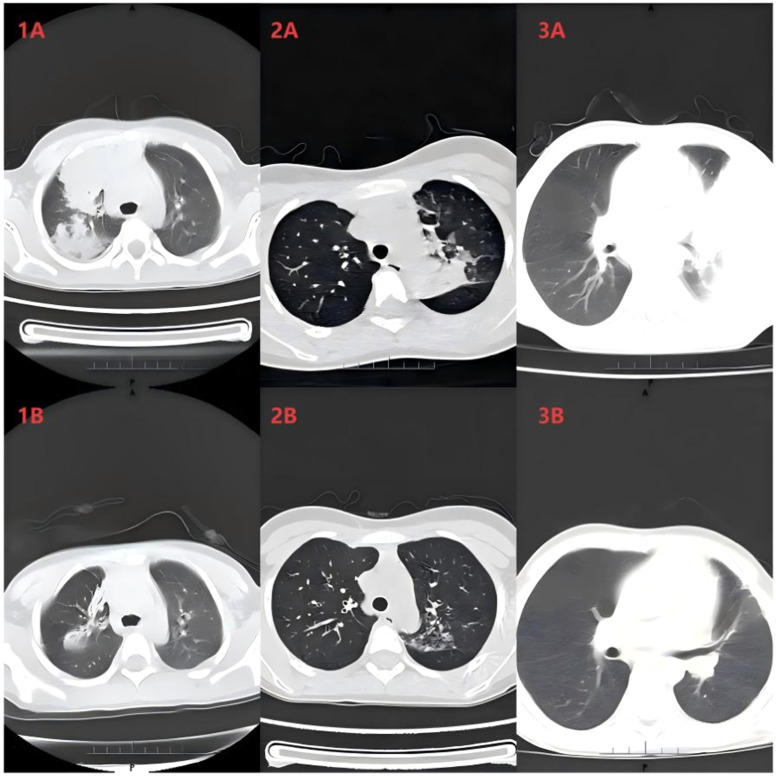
Findings on chest computed tomography before and after BAL. (1A) Case 1 (pre-BAL). (1B) Case 1 (2 weeks post-BAL). (2A) Case 2 (pre-BAL). (2B) Case 2 (1 week post-BAL). (3A) Case 3 (pre-BAL). (3B) Case 3 (2 weeks post-BAL). BAL, bronchoalveolar lavage
